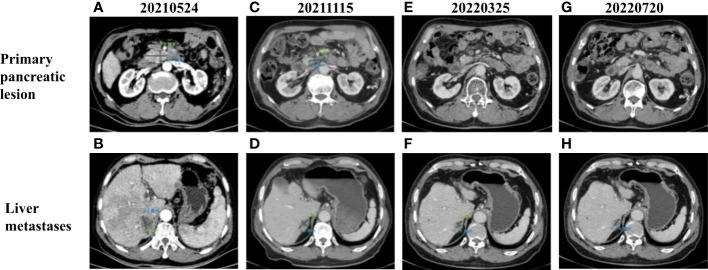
(A) CT images of primary pancreatic lesion with a size of 4.1 cm × 2 cm before treatment. (B) CT images of liver metastases with a size of 3.8 cm × 2.4 cm before treatment. Contrast-enhanced CT scan images completed in (C, D) November 2021, (E, F) March 2022, and (G, H) July 2022 demonstrating progressive decrease in the size of primary pancreatic and liver metastases.
